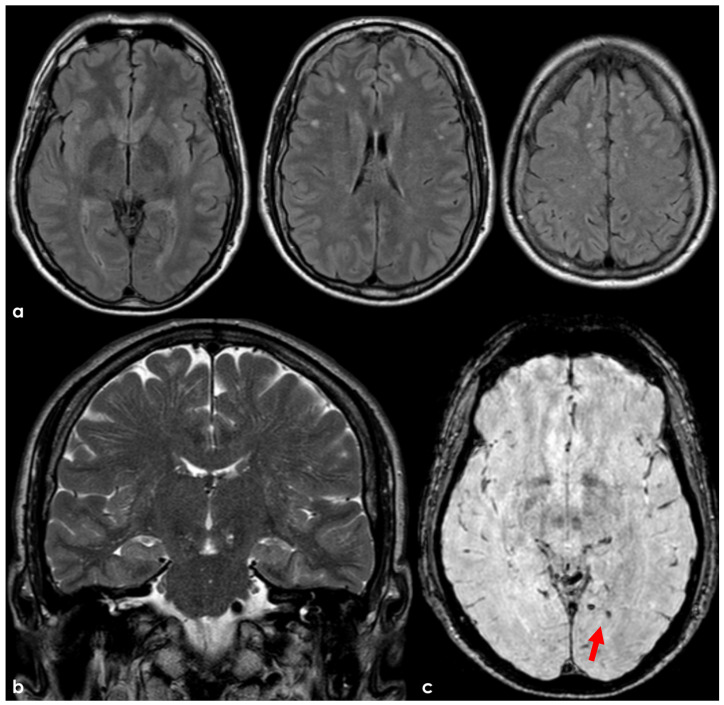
MRI of a patient fulfilling the diagnostic criteria for “possible CAA” and no restrictions for enrollment in Lecanemab and Donanemab treatment. Panel (a): axial FLAIR MRI showing a few punctate WMHs with a prevalent subcortical location, which resembles a WMH-MS. Panel (b): coronal T2W sequence with slightly enlarged perivascular spaces in the centrum semiovale. Panel (c): axial SWI with a single left temporal microbleed (red arrow).
